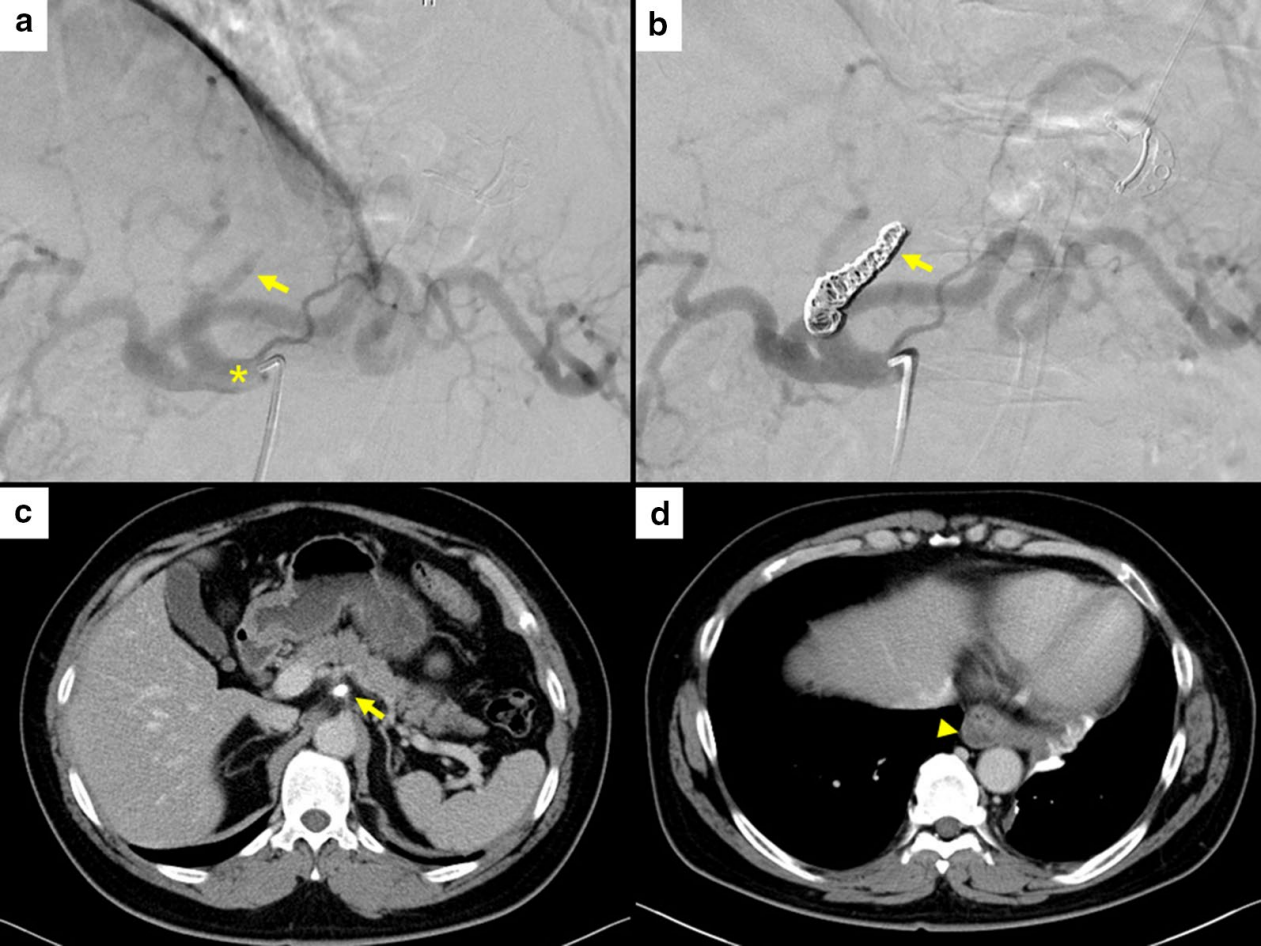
Interventional radiology and postoperative computed tomography images. a Celiac angiography (asterisk) showing slight residual blood flow in the aberrant artery (yellow arrow). b After coil embolization, the aberrant artery was found to be completely occluded (yellow arrow). c Computed tomography image showing occlusion of the aberrant artery at the celiac artery 6 months after surgery (yellow arrow). d Computed tomography image showing the shrunken remnant aberrant artery 6 months after surgery (yellow arrowhead)
